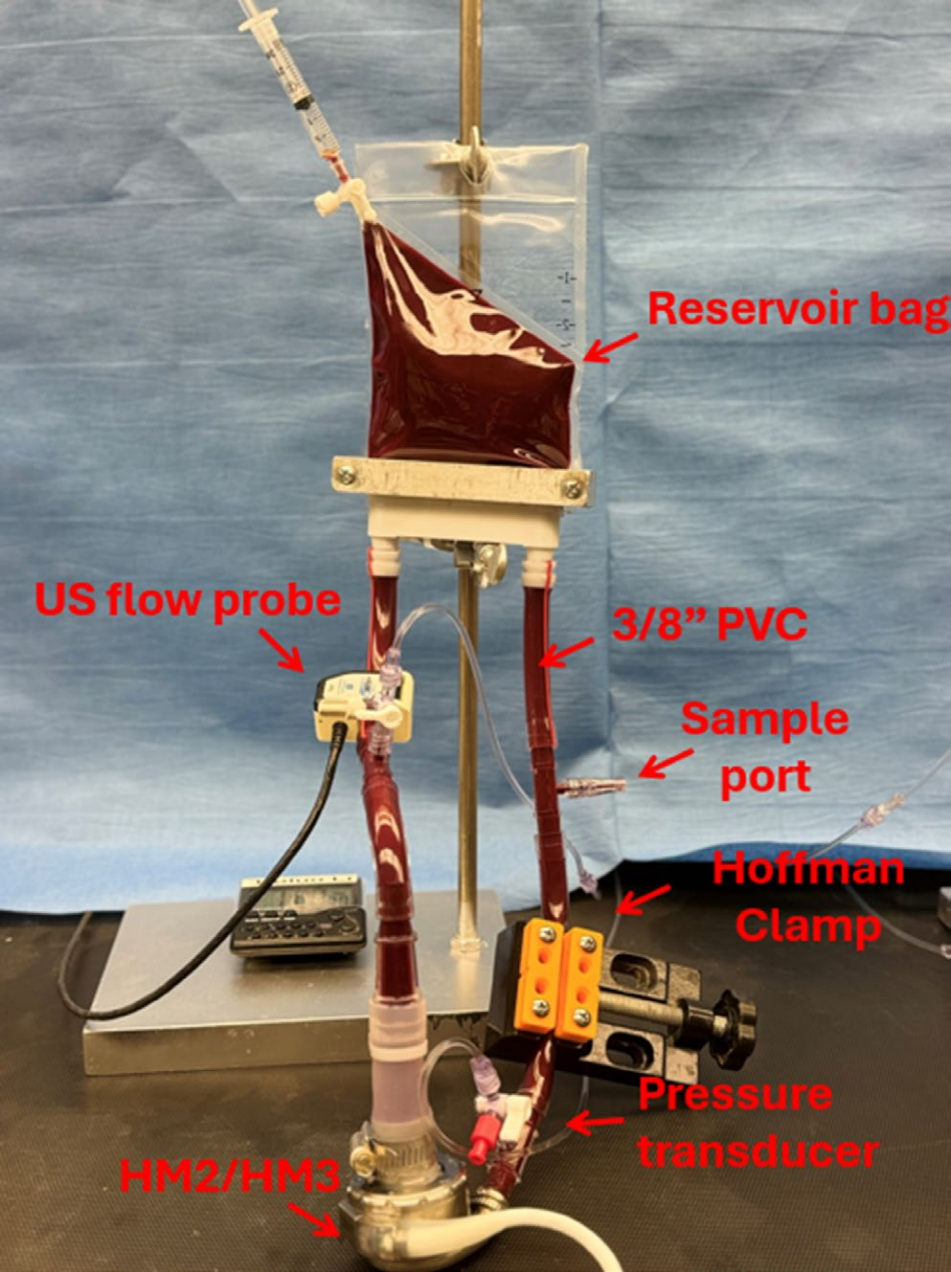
Experimental set‐up of the circulatory loop consisting of a blood reservoir, an HM2 or HM3 pump, polyvinylchloride tubing (3/8 in.), connectors, ports for blood sampling, a Hoffman clamp to adjust the pressure head, an ultrasound flow probe, and pressure transducers to measure the pressure pre and post the study pump. [Color figure can be viewed at wileyonlinelibrary.com]
